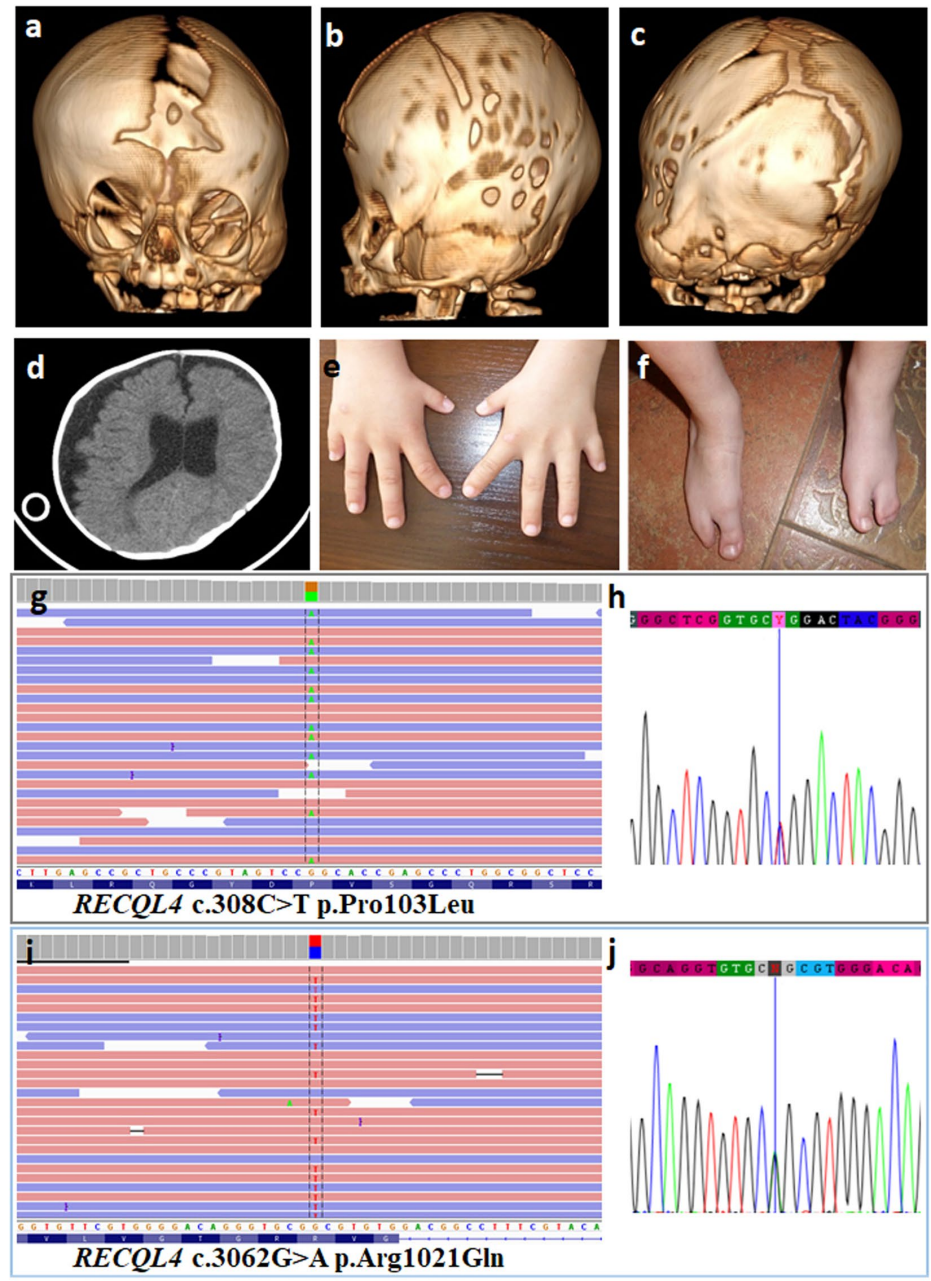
Clinical characteristics of Patient 15 at the age of 11 (a–d) and 9.5 years (e,f) as well as molecular results of the patient (g-j). Patient 15 presented with complex craniosynostosis composed of bilateral coronal synostosis (complete right-sided and partial left-sided) as well as partial left-sided lambdoid synostosis shown in 3D modelling of the skull (a–c). CT scan of the head (d). Coronal sutures are prematurely fused. The right coronal suture is completely fused (a), while the left one is only partially fused (b); consequently, there is marked enlargement of the anterior fontanelle and widening of the sagittal suture, (a,c). Asymmetry of the skull and brain, including lateral ventricles, and enlargement of left subarachnoid space seen on horizontal section (d). Limb defect clinically recognized as bilateral split foot malformation with syndactyly of the remaining toes, extremely short and hypoplastic thumbs and 5^th^ fingers, short 5^th^ metacarpals and valgus deformity of the right 2^nd^ finger (e,f). Representation of the compound heterozygous *RECQL4* deleterious variants c.308C > T p.Pro103Leu and 3062G > A p.Arg1021Gln detected in Patient 15 by means of targeted next-generation sequencing (g,i). Both pathogenic variants were confirmed with the use of Sanger sequencing (h,j).
